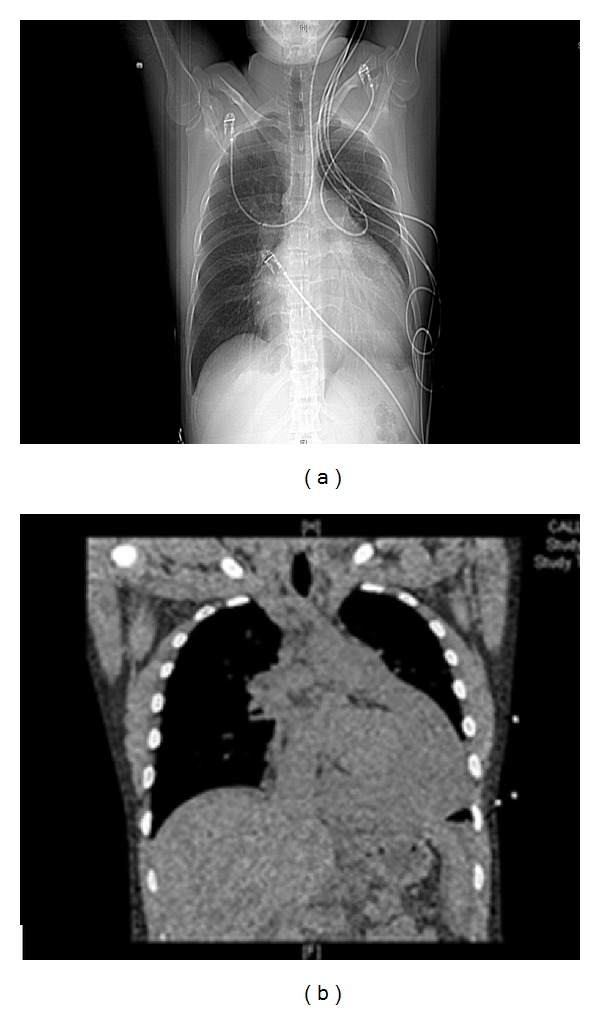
Radiographic imaging; chest X-ray (a) and CT scan (b).
